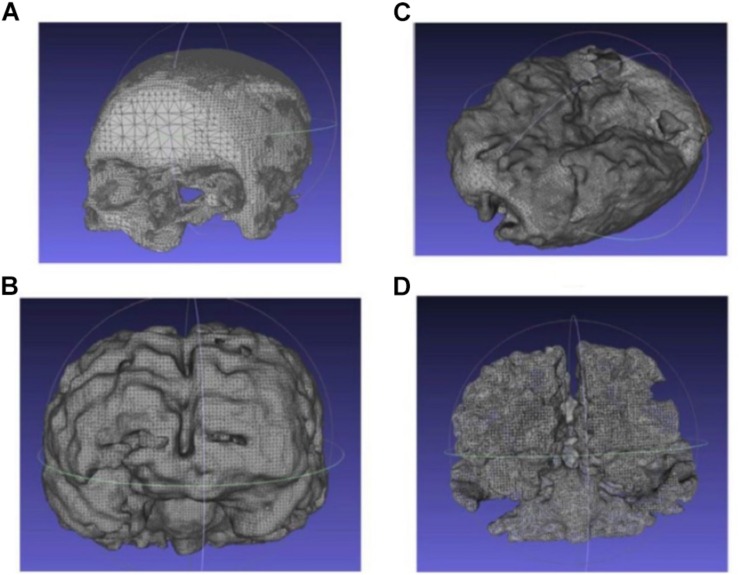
Extracted and processed tissue models from the MRI image stack (A) skull, (B) CSF, (C) gray matter, and (D) white matter.
